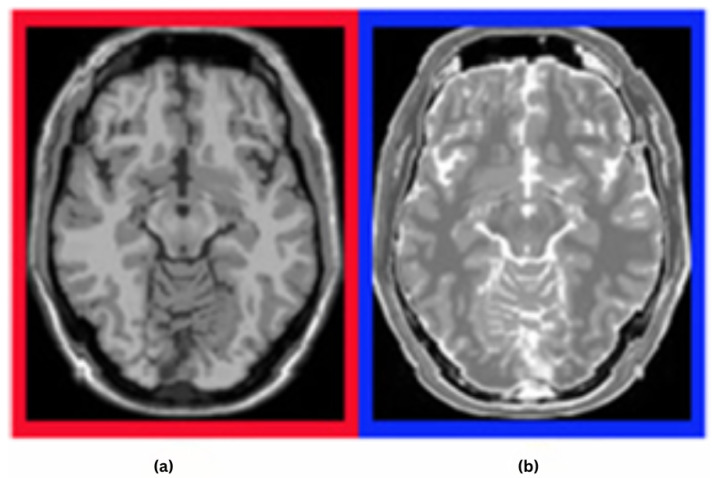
Examples of MRI sequences of an MS brain: (a) T1- and (b) T2-weighted images [3].
